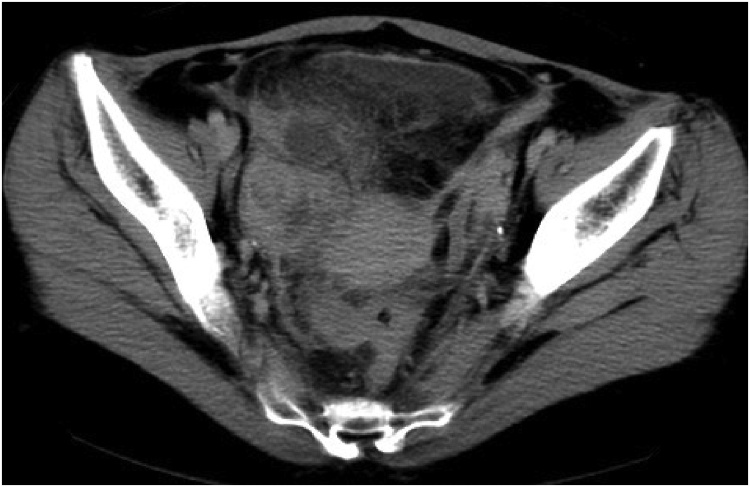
CT revealed an abscess at the right lower quadrant.
